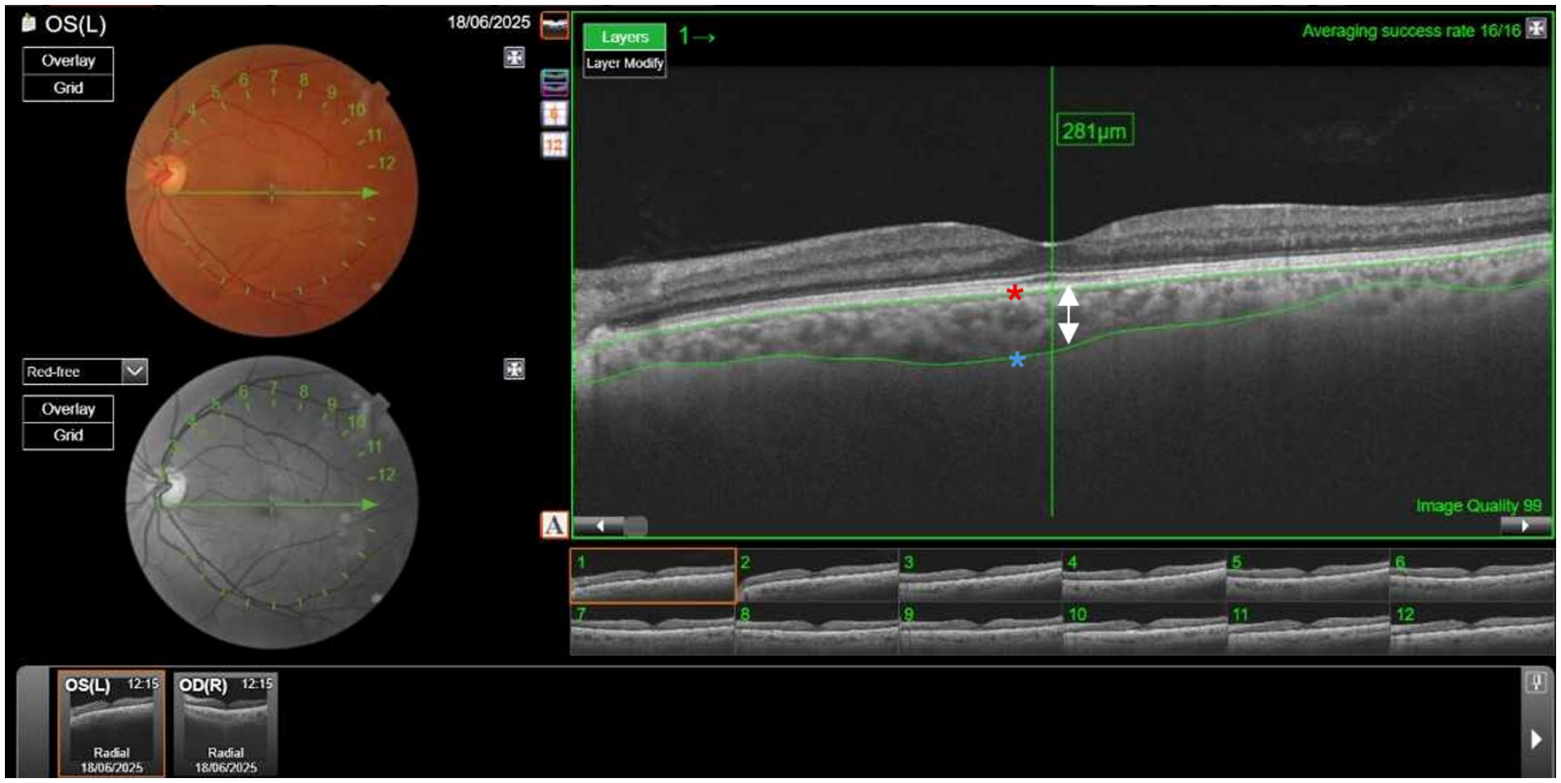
Measurement of choroidal thickness (CT) using Topcon DRI-OCT Triton Swept-Source-Optical coherence tomography (SS-OCT). Subfoveal CT was defined as the distance from the outer reflective line of the retinal pigment epithelium (red asterisk) to the inner limit of the sclera (blue asterisk). The arrow shows the thickness of the choroid layer, in this case, 281μm. (Picture provided by Dra. Esteban-Ortega, Ophthalmology service).
